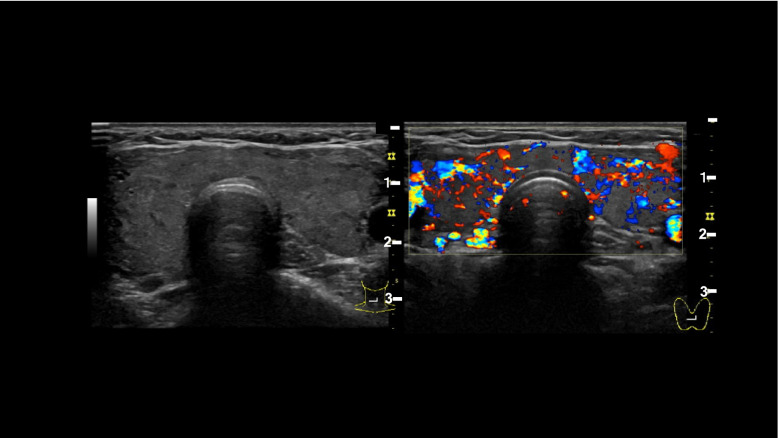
Thyroid ultrasonography. Thyroid ultrasonography revealed diffuse swelling of the thyroid gland and abundant blood flow
